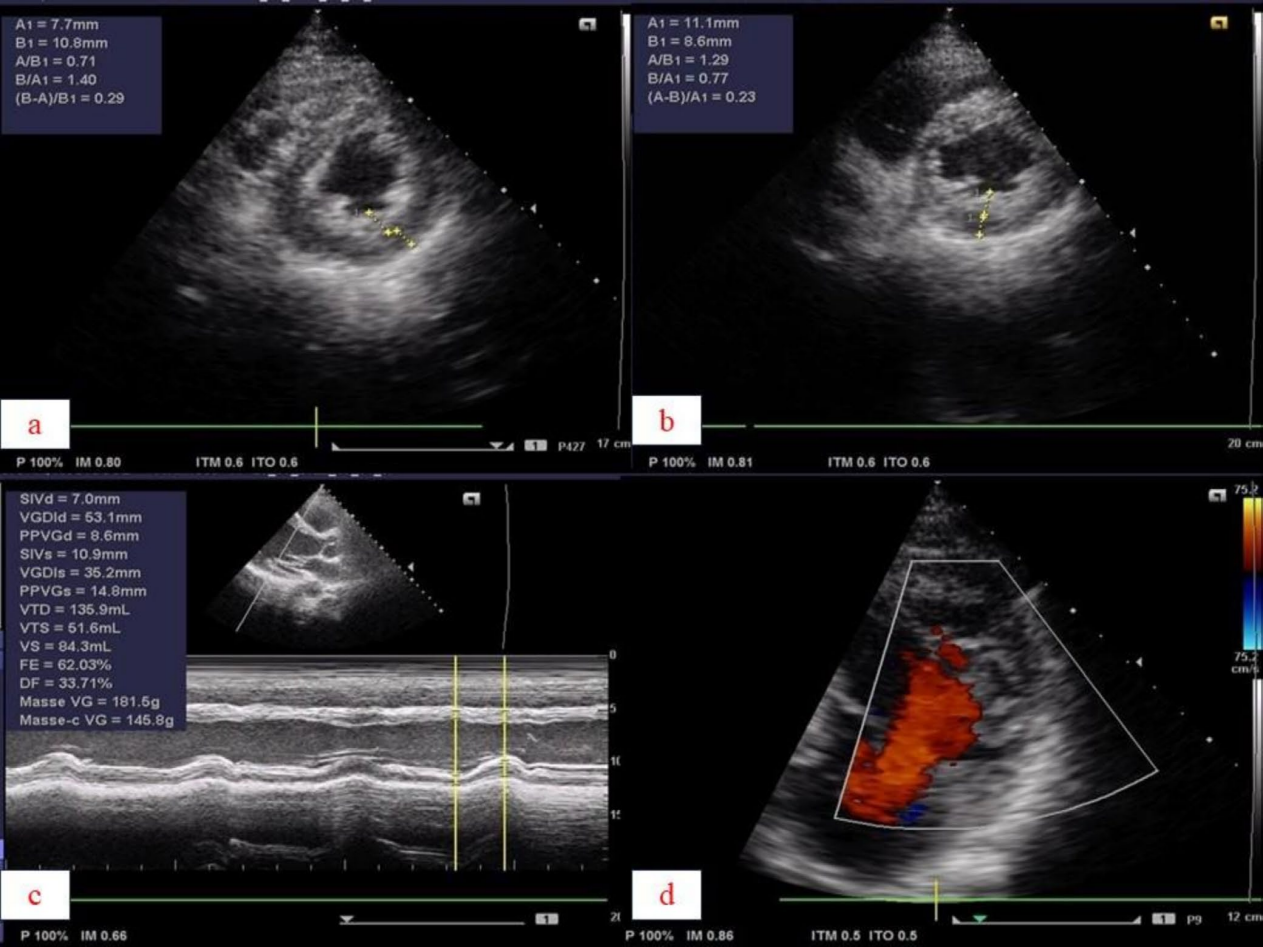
Echocardiography showing numerous trabeculations in the left ventricle. (a) Short‐axis asternal section showing a ratio of non‐compacted area to compacted area = 2 measured at the side wall. (b) Short‐axis asteroidal section showing a ratio of non‐compacted area to compacted area = 1.8 measured at the lower wall. (c) Long‐axis asternal section showing a FeVG calculated with Teicholz at 62%. (d) Color Doppler showing a vascularised recess inside the left ventricle.
